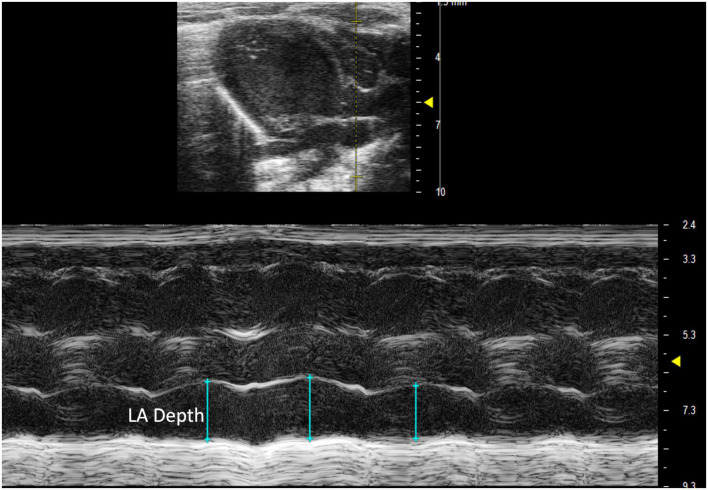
Parasternal long axis (PLAX) M-mode view displaying left atrial depth measurement just inferior to the aorta.
